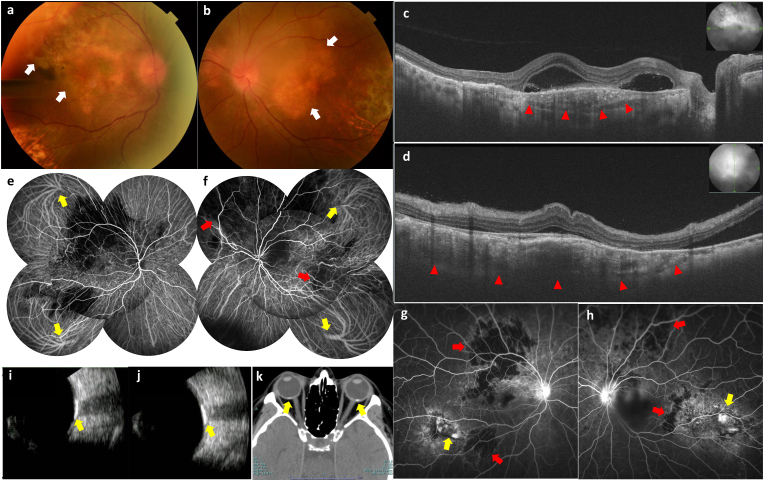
Color photographs, posterior segment optical coherence tomography (PS-OCT) images, indocyanine green angiography (ICGA) images, fluorescence angiography (FA) images, Ultrasonographic images, and computed tomography (CT) images of bilateral choroidal osteoma. (a, b) Color photographs of the right eye (a) and the left eye (b) showing the yellowish to orange, slightly elevated subretinal lesion (black arrows). (c) Horizontal PS-OCT images of the right eye with subretinal fluid and a mass under retinal pigment epithelium (RPE) (red arrowheads). (d) Vertical PS-OCT images of the left eye with subretinal fluid and a mass under RPE (red arrowheads). (e, f) Early-phase ICGA images of the right eye (e) and the left eye (f) showing partial hypocyanine choroidal circulation (red arrows) and dilated choroidal vortex veins (yellow arrows). (g, h) intermediate-phase FA images of the right eye (g) and the left eye (h) showing hypofluorescent spots (red arrows) and hyperfluorescent spots (yellow arrows). (i, j) Ultrasonographic images of the right eye (i) and the left eye (j) showing hyperechogenic mass at the posterior wall of both eyes with deeper acoustic shadows (yellow arrows). (k) CT showing calcification of the choroid at the posterior wall of both eyes (yellow arrows). (For interpretation of the references to colour in this figure legend, the reader is referred to the Web version of this article.)
